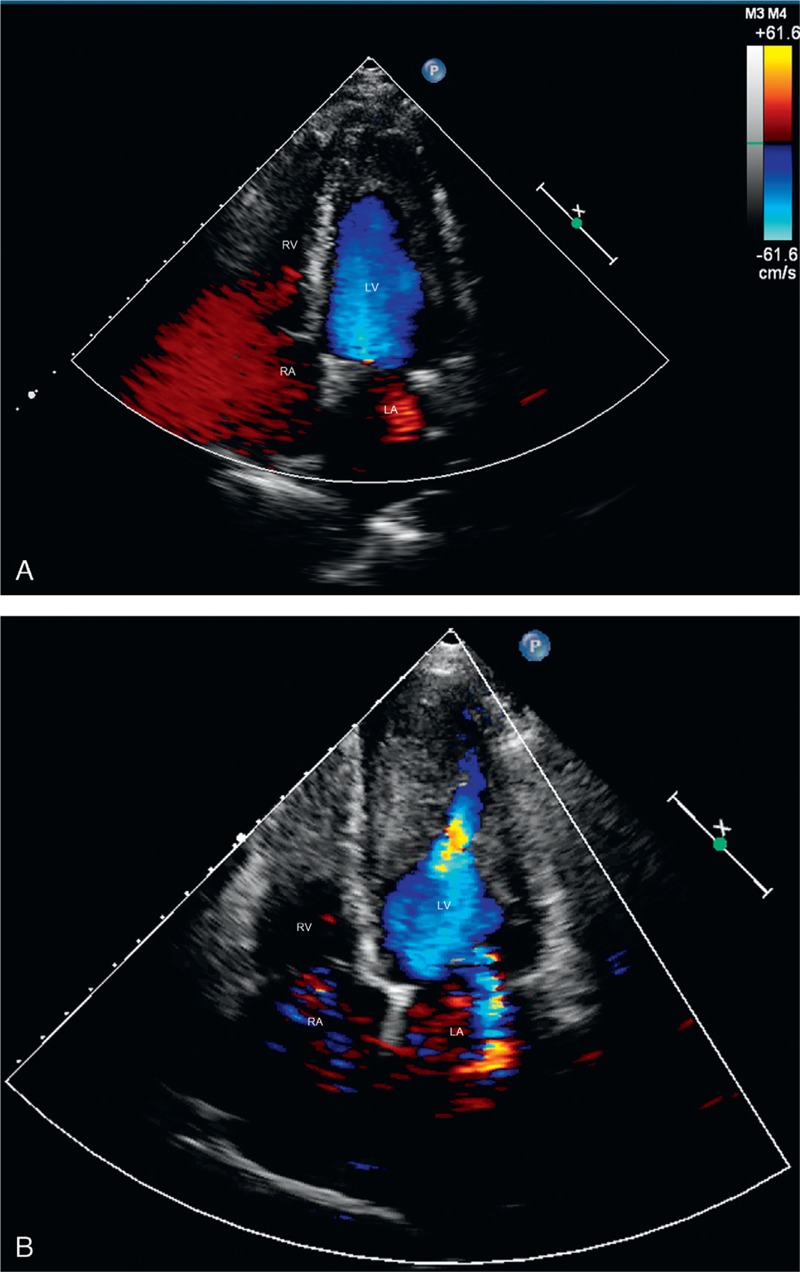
Images of 2D TTE examination of the second patient. Image (A) shows cap-shaped echoes of a mural thrombus at the apex and middle part of the LV cavity. Image (B) shows that at 5 month follow-up, the size of the thrombus was increased substantially whereas blood flow could be found in the central thrombus during the diastole phase. 2D = 2-dimensional, LV = left ventricular, TTE = transthoracic echocardiography.
